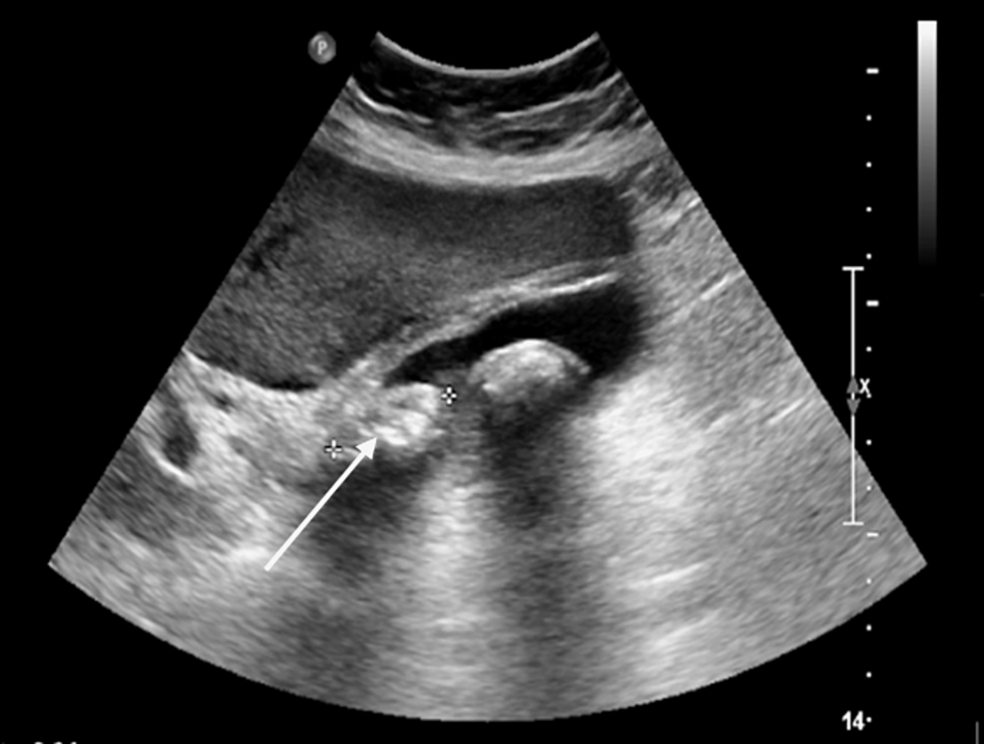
Ultrasound image shows gallstone, gallbladder thickening, pericholecystic fluid, and sonographic Murphy’s sign Arrow indicates gallstone
